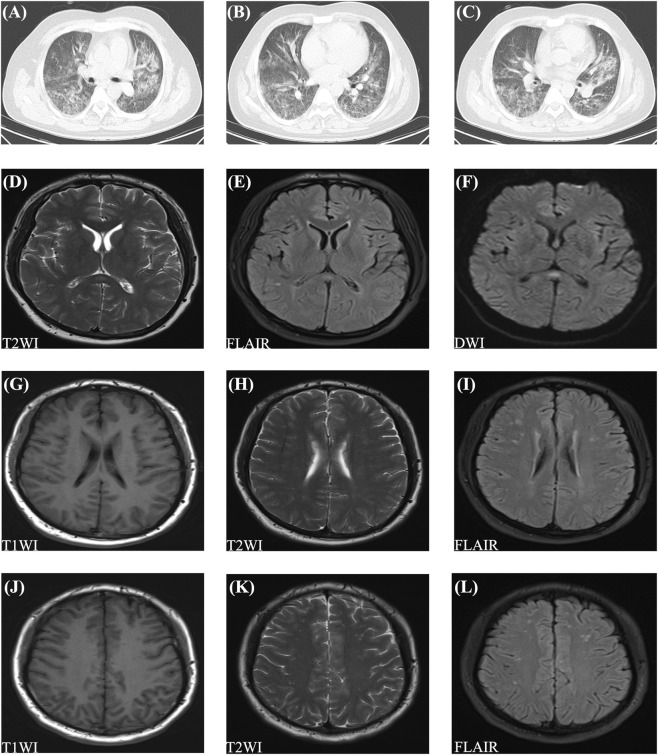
Chest CT and cranial MRI scans of the patient. (A–C) Chest CT showing patchy and flocculent opacities in both lungs. (D–F) Cranial MRI demonstrating nodular hyperintense on T2WI, FLAIR and DWI in the splenium of corpus callosum. (G–I) Cranial MRI showing multiple patchy hypointense on T1WI, and hyperintense on T2WI and FLAIR around periventricular. (J–L) Cranial MRI showing multiple patchy hypointense on T1WI, and hyperintense on T2WI and FLAIR around semioval center. CT: computed tomography; MRI: magnetic resonance imaging; T2WI: T2-weighted imaging; FLAIR: fluid attenuated inversion recovery; DWI: diffusion-weighted imaging; T1WI: T1-weighted imaging.
